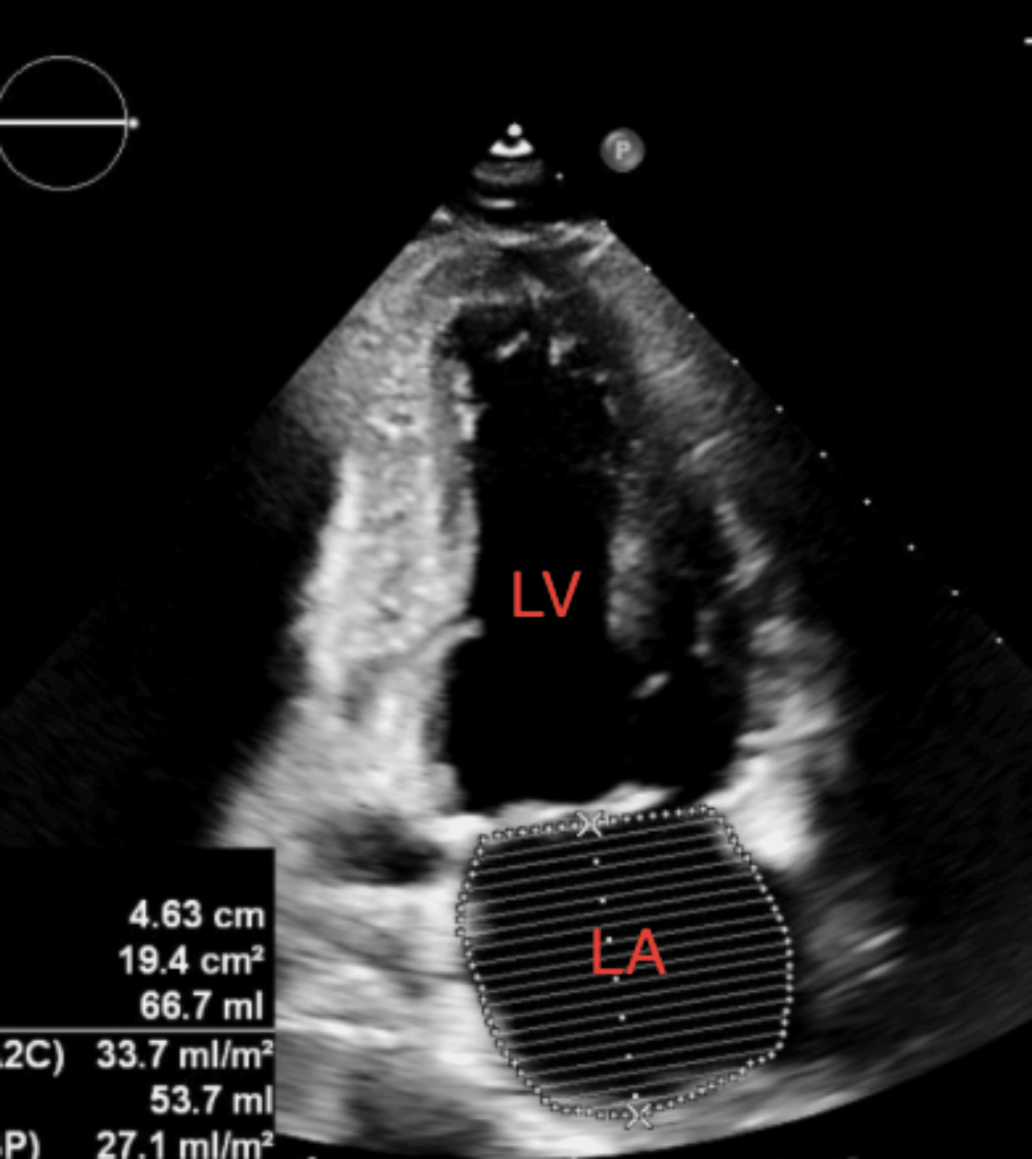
Transthoracic echocardiogram four months after discharge, showing left ventricular ejection fraction of 55%. Two-dimensional apical chamber view. Right-sided chambers are not well visualized. The dotted chamber has no significance. Imaging came from an external facility, and the authors did not have access to the original study. The image above is the only echocardiogram in the chart for this visit. LA: left atrium; LV: left ventricle.
